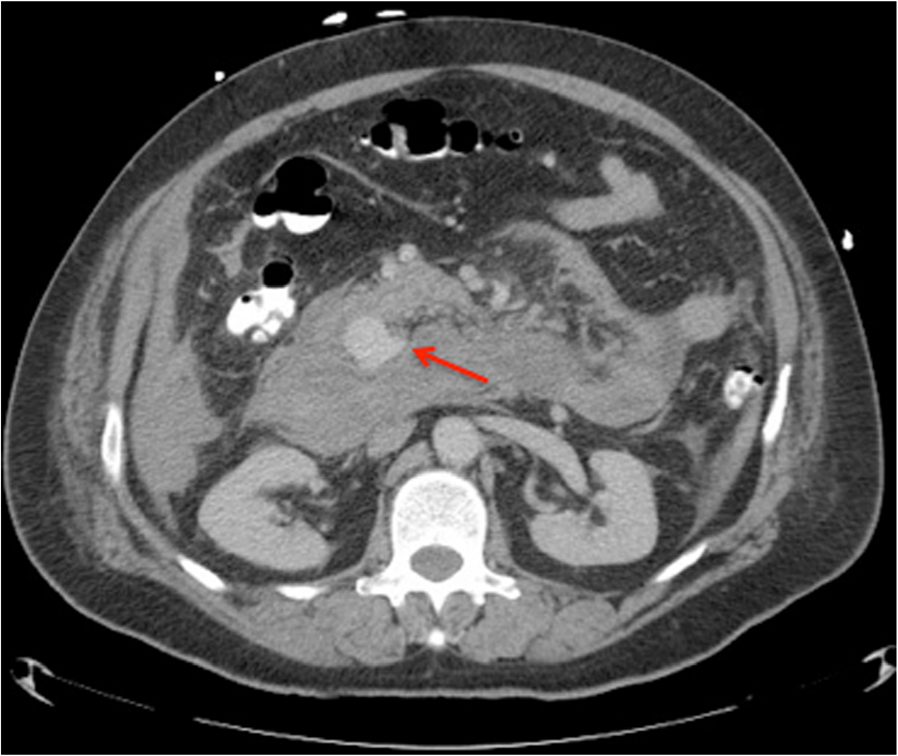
Abdominal contrast enhanced computed tomography reveals retroperitoneal aneurysm (arrow) that is suspected to be arising from the gastroduodenal artery or one of its branches.
